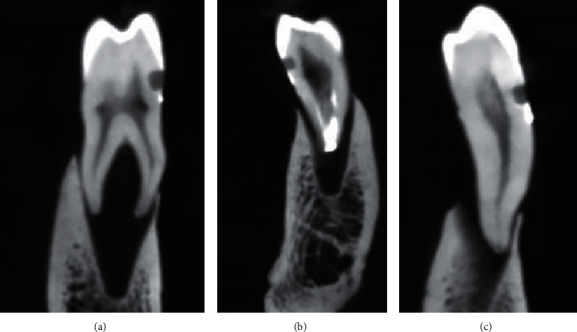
Marginal leakage on the gingival wall detected by the New Tom CBCT unit (New Tom GiANO; Quantitative Radiology, Verona, Italy). (a) Score 0. (b) Score 1. (c) Score 2.
